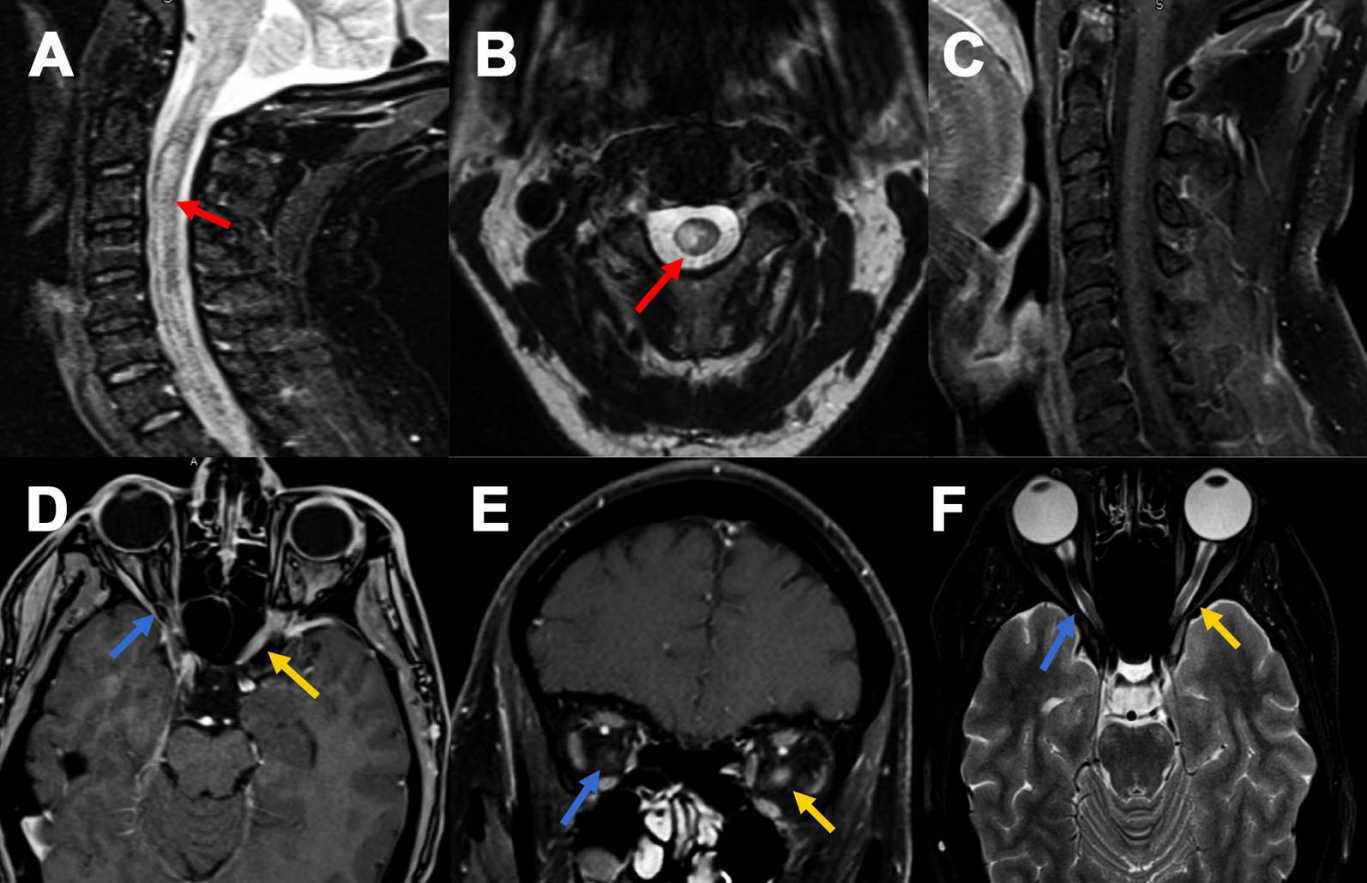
MRI sagittal STIR images (A) of the cervical spine reveal ill-defined long segment hyperintensity with prominent cord expansion C2–C4 (red arrow); B shows axial cut with cord signal alteration at C3 (red arrow), and C post-contrast sagittal image showed no abnormal enhancement. MRI orbit Axial T1-weighted fat suppression post-contrast (D) and coronal images (E) reveals abnormal enhancement of left optic nerve pre-chiasmatic (intracanicular); (yellow arrow) with corresponding hyperintense on axial T2 weighted images (F) (yellow arrow) with no abnormality of the right optic nerve (blue arrow)
